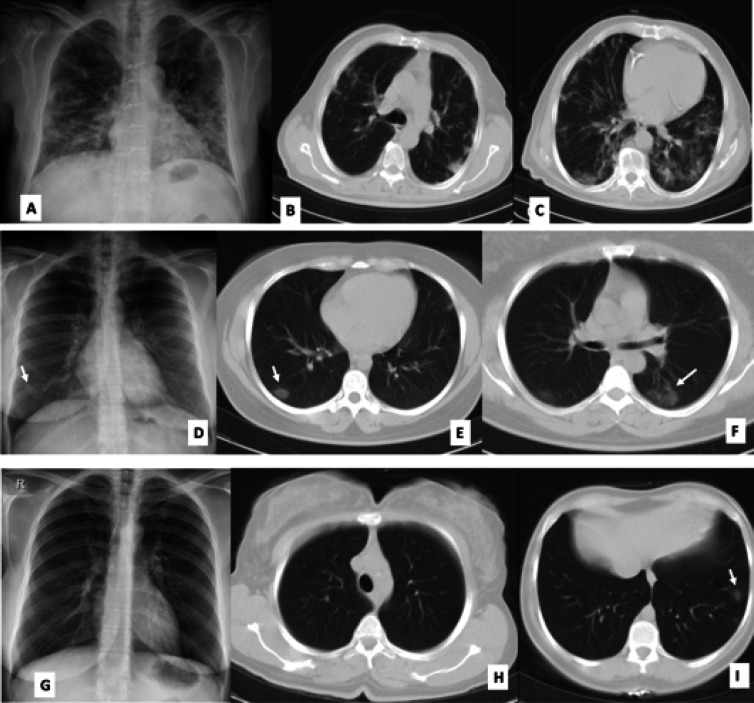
Variety of chest graph changes in patients with confirmed COVID-19 pneumonia. In patient 1, radiograph shows multifocal patchy airspace opacifications in both lungs, more severe in the mid and lower zones of the lungs (A; arrows). CT shows peripheral ground-glass opacities and consolidations in the upper and lower lobes (B&C). In the second patient, radiograph (D) shows a subtle opacity in the right lower zone corresponding to peripheral ground-glass opacity in the right lower lobe (arrow; E). The opacification in the left lower lobe (F) is not visible on the radiograph. In patient 3 (G) the radiograph was normal and missed small opacity in the left lower lobe (I)
